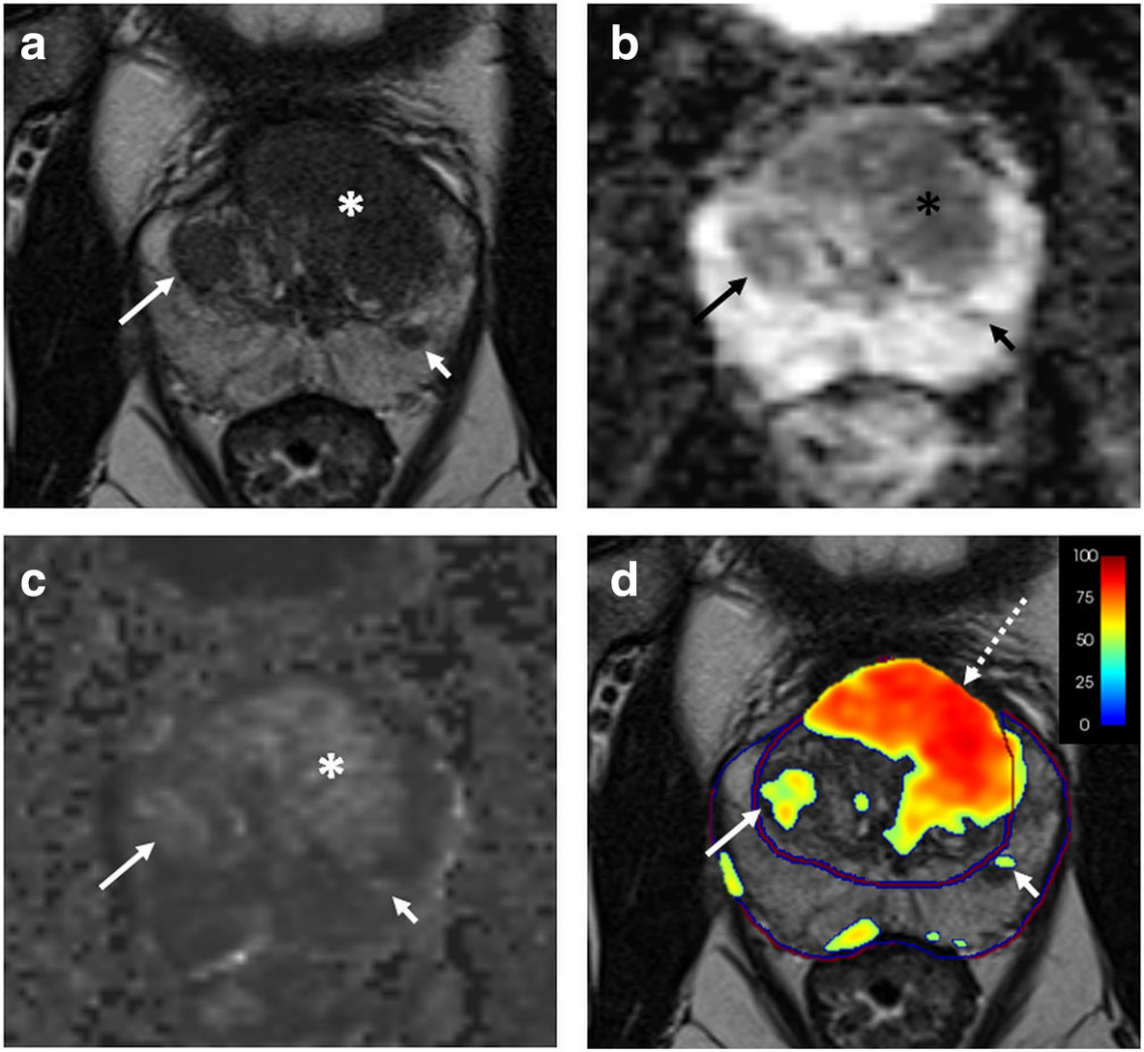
A 66-year-old man with a serum PSA of 14.3 ng/mL. Axial T2-weighted imaging (a), ADC map (b), and calculated *b* = 1500 s/mm^2^ image (c) showed a lesion in the left mid TZ (asterisk in a, b, c). In addition, note a BPH nodule in the right mid TZ (long arrow in a, b, c) and an ectopic BPH nodule in the left mid PZ (short arrow in a, b, c). Computer-aided diagnosis map overlaid on the T2-weighted image (d) showed a high cancer probability within the left mid TZ lesion (dashed arrow), whereas it showed low cancer probability within the right mid TZ BPH nodule (long arrow) and the ectopic BPH nodule in the left mid PZ (short arrow). Final pathology revealed a Gleason 7 (3 + 4) prostate cancer within the left mid TZ lesion (image courtesy of Dr. Baris Turkbey and Dr. Stephanie Harmon from Molecular Imaging Program, NCI, NIH, Bethesda, MD, USA)
